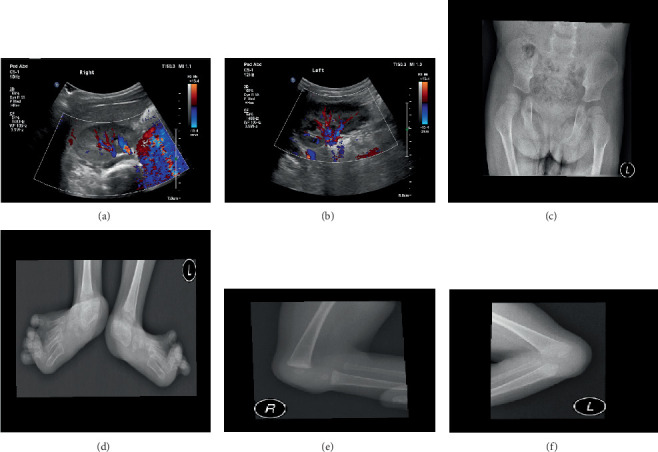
Multiple diagnostic radiographs. (a, b) Ultrasound for the kidney during pregnancy shows hydronephrosis. (c–f) Severe clubbing to the foot and suspected partial metatarsus varus deformity of the left foot with hallux valgus of the first toe, as well as metatarsus varus and clubfoot of the right foot.
